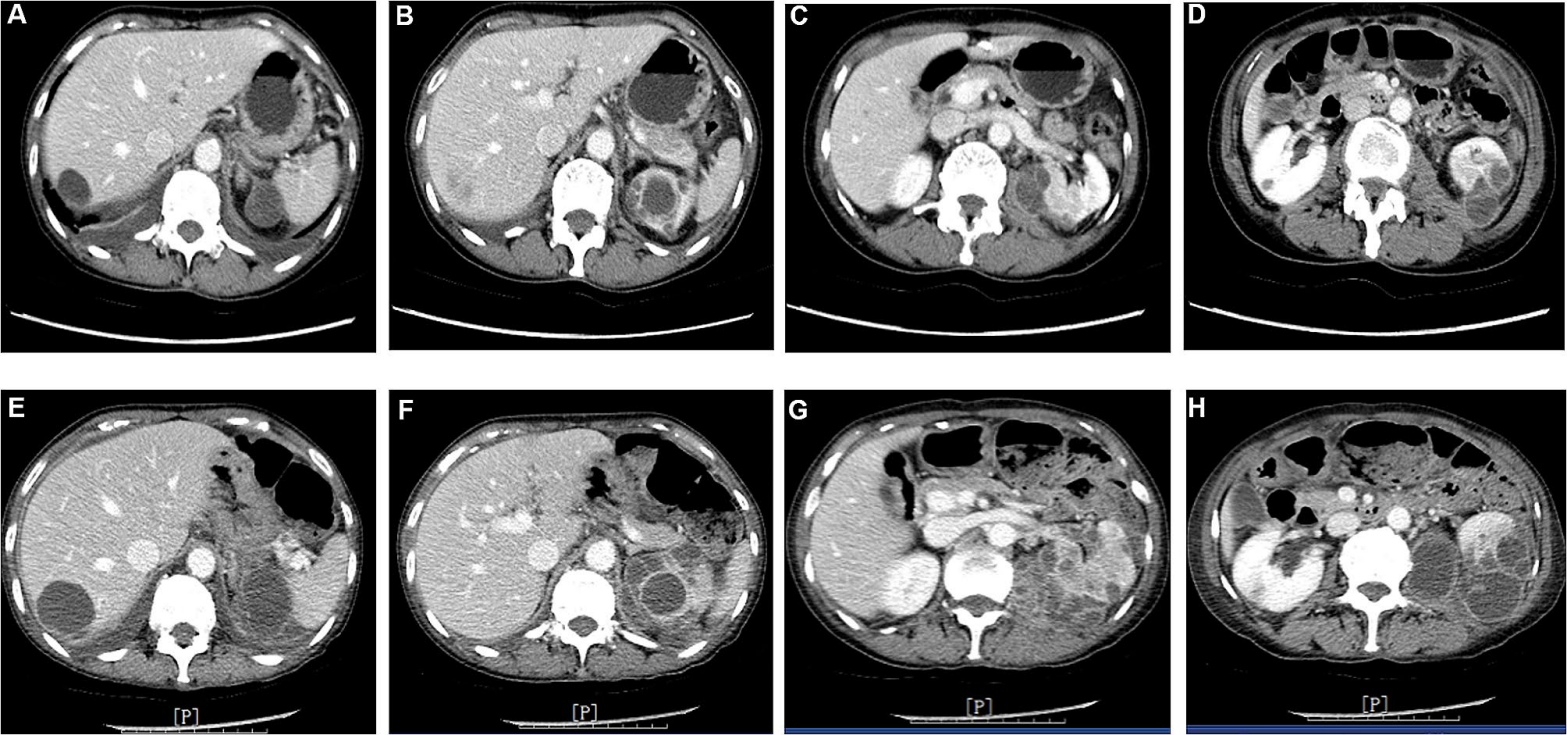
Contrast-enhanced abdominal CT scan during crizotinib treatment. Panels A-D: Post 3 months on crizotinib treatment, demonstrating the cystic lesions have been developed in the kidneys and liver. Panels E-H: Post 5 months on crizotinib treatment, demonstrating the cystic lesions in the kidneys and liver continuously grew during the additional 2 months of crizotinib treatment
